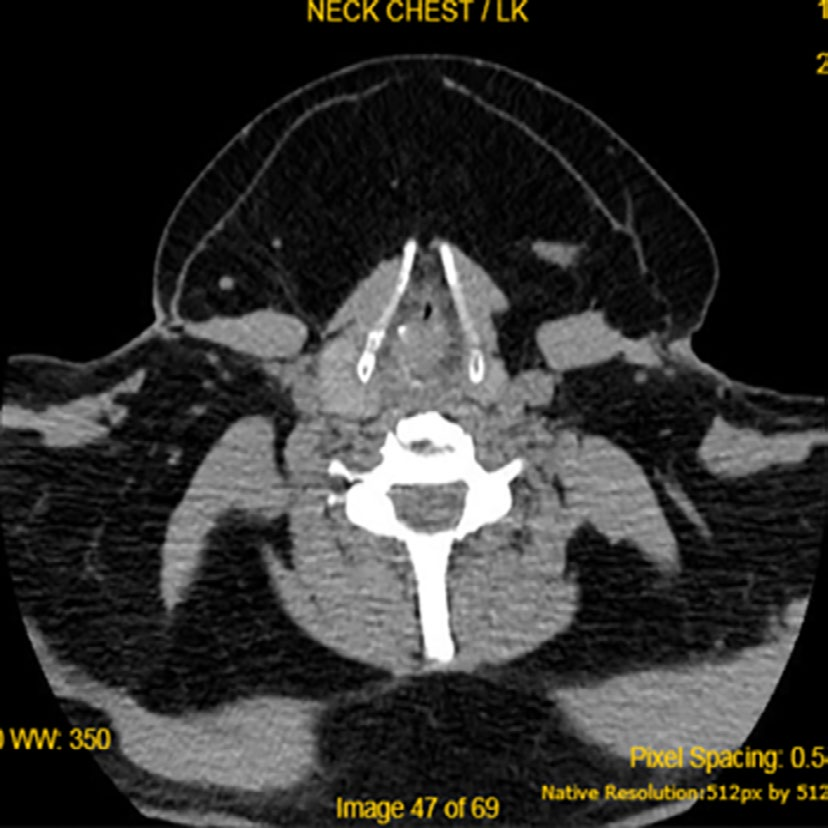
Diffuse lipomatosis on CT scan
